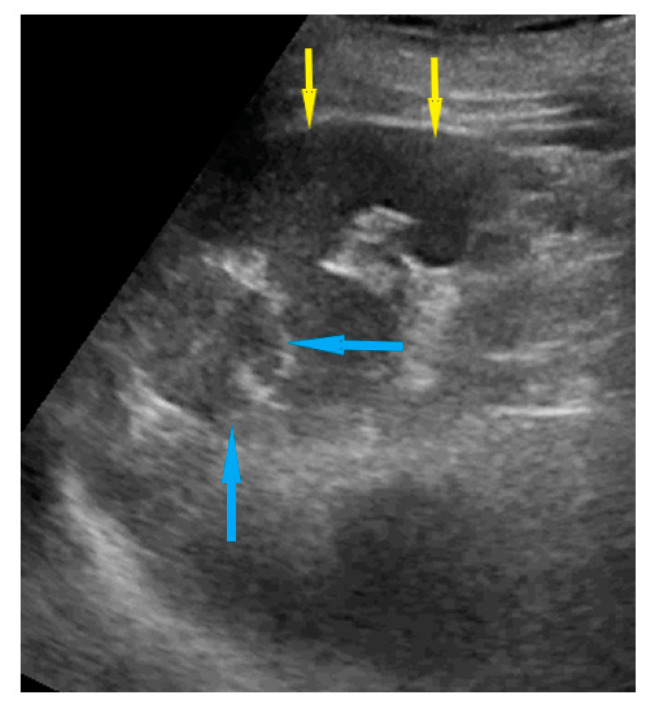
Ultrasound image of spleen parenchymal lesions (blue arrows) (yellow arrows identify normal spleen parenchyma).
